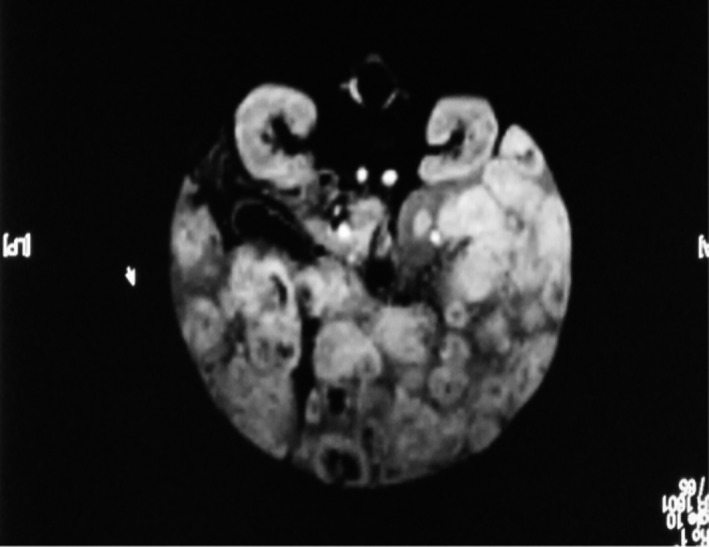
MRI of the abdomen revealing huge heterogeneous hepatomegaly with multiple diffuse intrahepatic well‐defined nodular structures (1–4 cm of diameter) with low signal intensity on T1 and high on T2. There was peripheral enhancement on the arterial phase and homogenization at the delayed venous phase.
